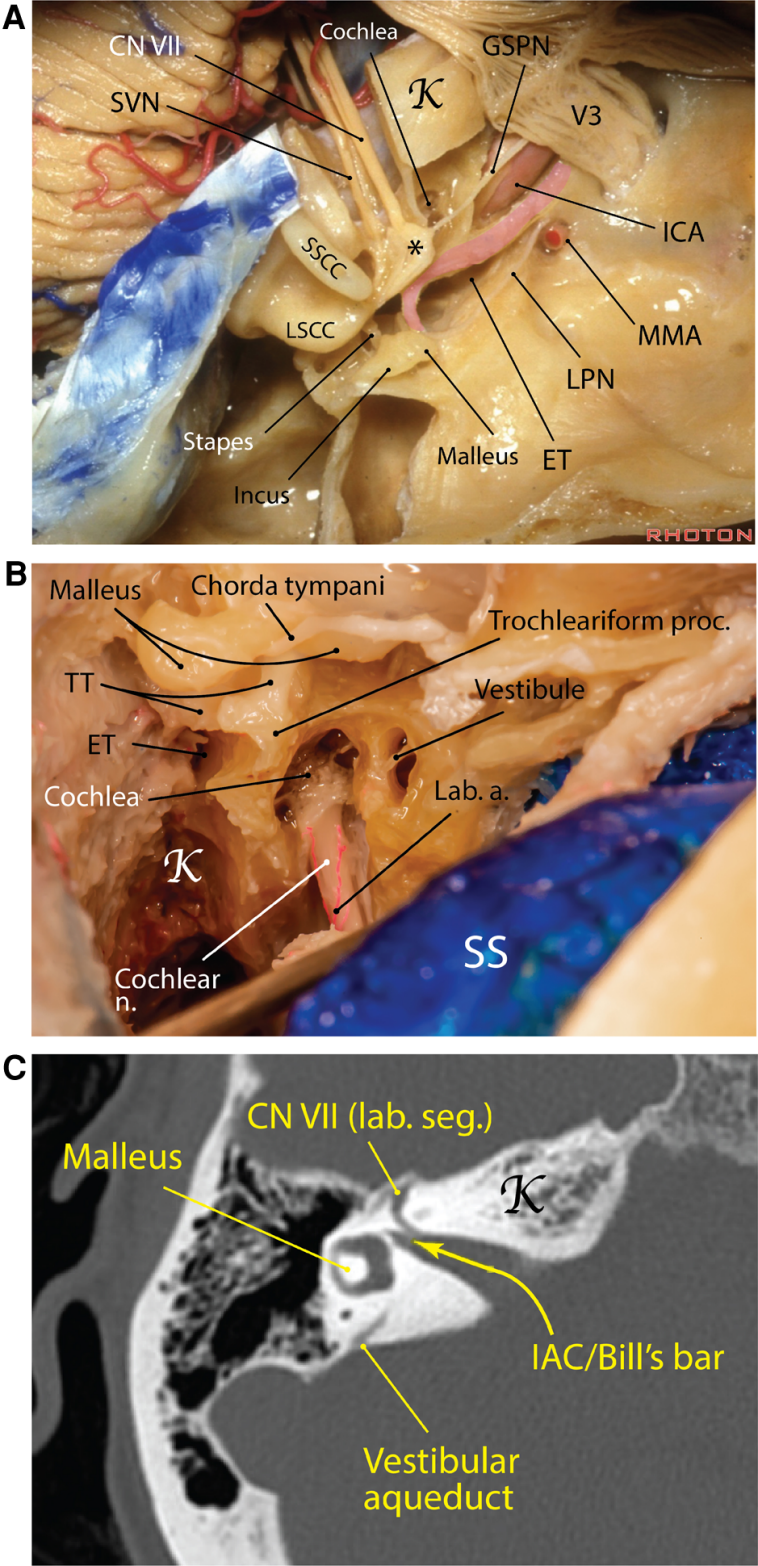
Carotid canal Eustachian tube in the middle cranial fossa. (A) The carotid canal starts at the exocranial surface of the temporal bone anteromedial to the jugular foramen. The GSPN runs on top of the petrous ICA and a thin shell of bone may separate the two. The Eustachian tube runs parallel and lateral to the carotid canal and harbors the tensor tympani muscle (pink area). *Geniculate ganglion [Courtesy of *The Rhoton Collection*, American Association of Neurological Surgeons (AANS)/Neurosurgical Research and Education Foundation (NREF)]. (B) A right mastoidectomy exposing the middle ear structures and the posterior end of the Eustachian tube, which is adjacent to the trochleariform process (Courtesy of Barrow Neurological Institute. Used with permission). (C) Right temporal bone computed tomography showing the internal auditory canal and the vertical crest (aka Bill's bar). a., artery; CN, cranial nerve; ET, Eustachian tube; GSPN, greater superficial petrosal nerve; ICA, internal carotid artery; K, Kawase's rhomboid; lab., labyrinthine; LPN, lesser petrosal nerve; LSCC, lateral semicircular canal; MMA, middle meningeal artery; n., nerve; proc., process; seg., segment; SS, sigmoid sinus; SSCC, superior semicircular canal; SVN, superior vestibular nerve; TT, tensor tympani.
